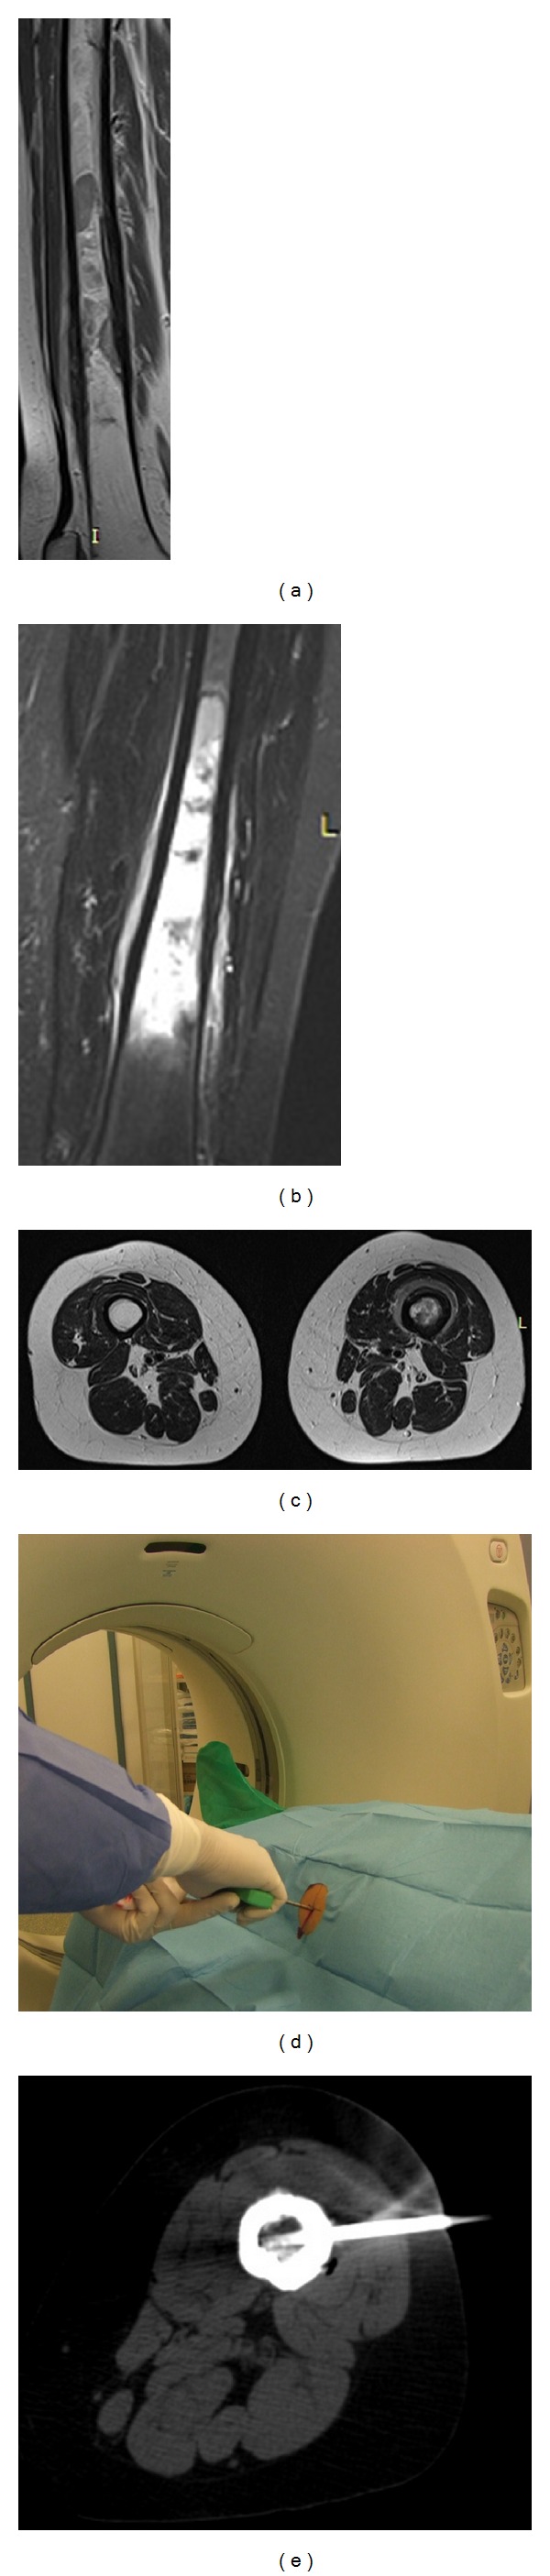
Sagittal MRI T1 (a), coronal MRI STIR (b), and axial MRI T2 (c) scans of the right thigh show heterogeneous intratumoral hypointensive signal on T1 and hyperintensive signal on T2 in the diaphysis of the femur. An 8-gauge core-needle bone biopsy inserted under CT guide (d) into marrow of the right femur (e). Diagnosis: Lymphoma.
